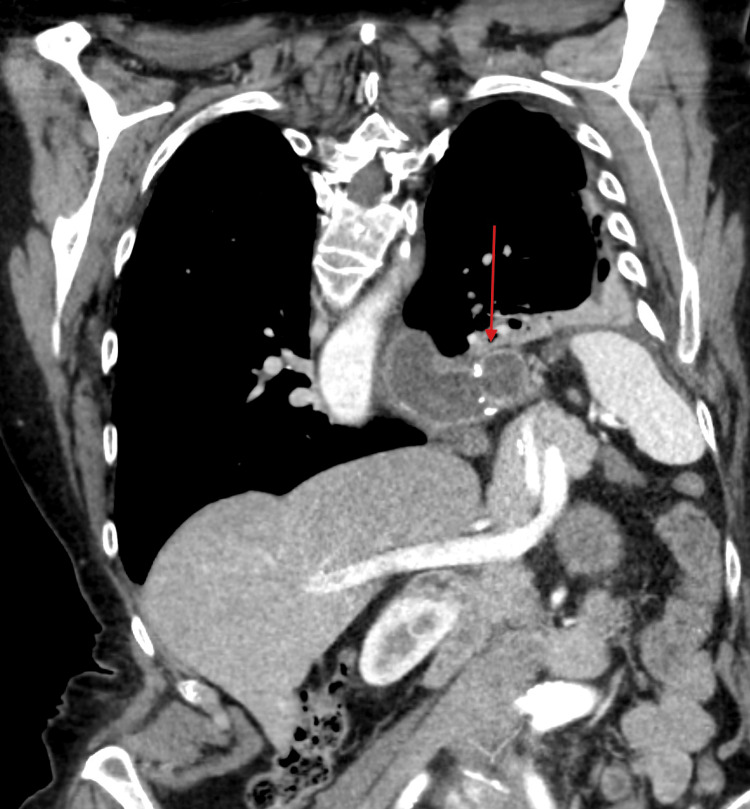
Coronal contrast-enhanced CT image obtained postoperatively demonstrating the intrathoracic esophagogastric anastomosis (red arrow) The anastomotic site appears intact, with no evidence of contrast extravasation, suggesting the absence of leakage.
